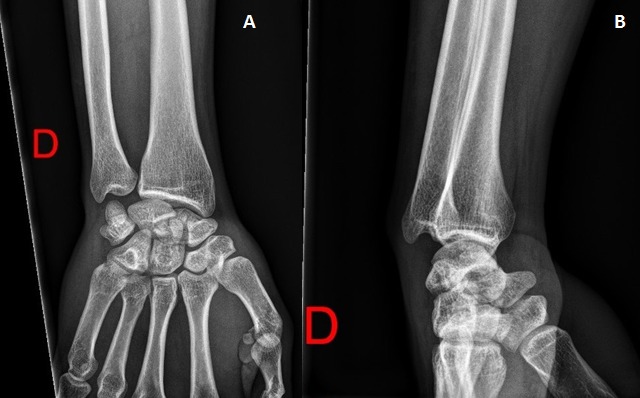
Radiographie du poignet droit de face et de profil: fracture transtuberculaire du scaphoide carpien associée à une fracture du capitatum réalisant le syndrome de Fenton (A,B)
